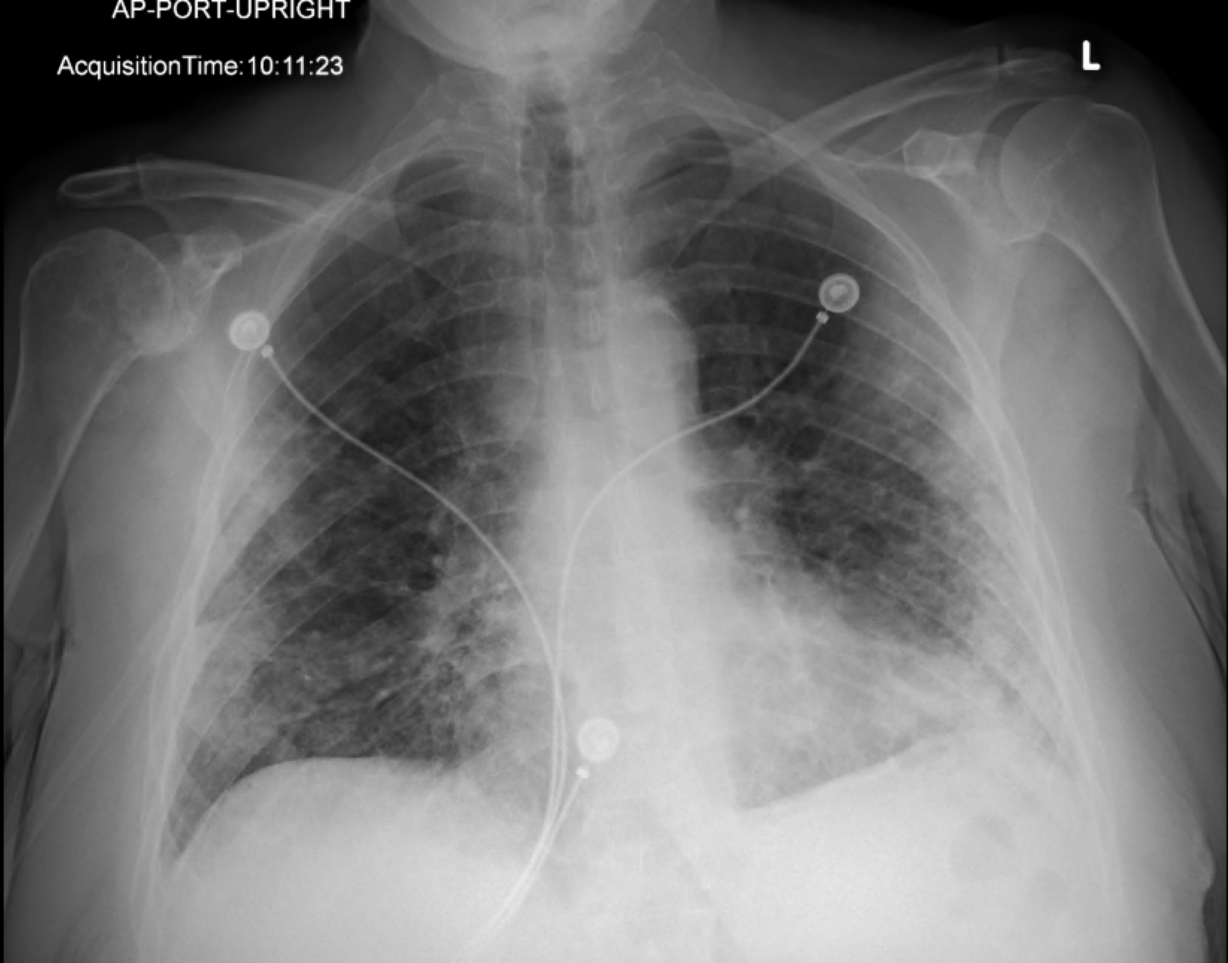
Repeat chest X-ray showing persistent multifocal airspace disease with interval worsening in aeration.
